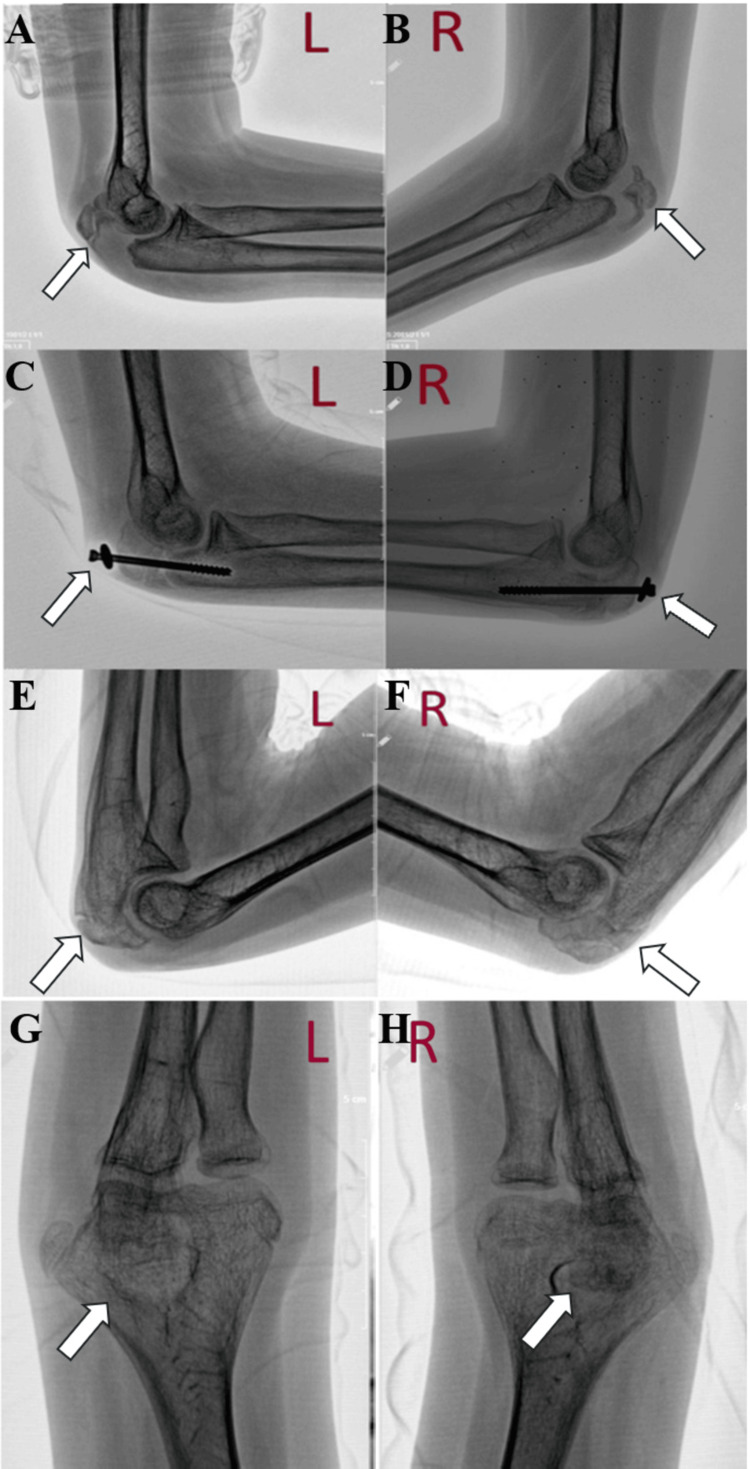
(A, B) Initial lateral plain radiographs of both the right and left elbows taken in the emergency room. (C, D) Lateral plain radiographs of both elbows at six weeks of follow-up. (E, F) Lateral radiographs of both elbows at one year of follow-up. (G, H) Both elbows' anteroposterior (AP) radiographs at one year of follow-up.
